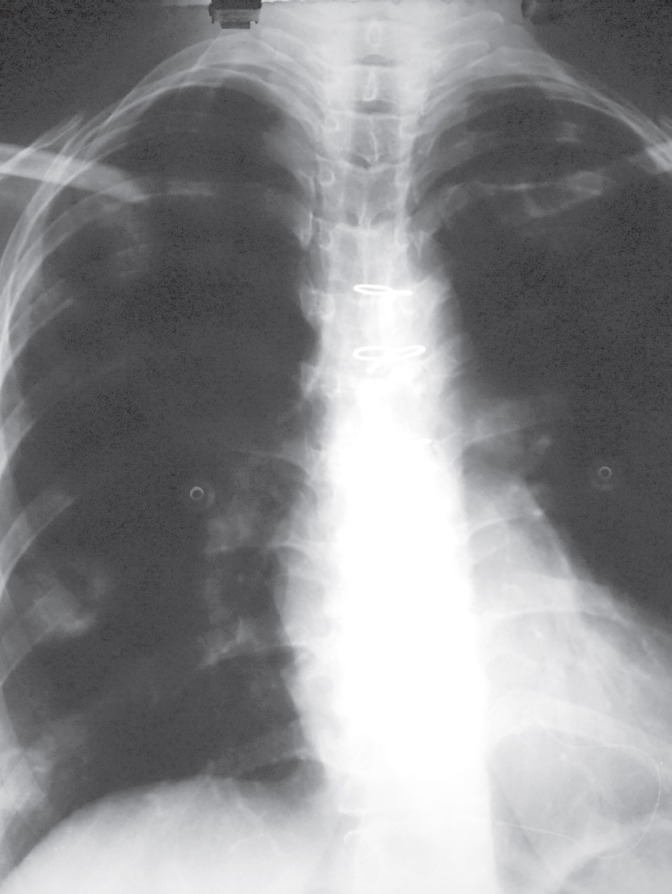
Normal chest x-ray at the time of initial presentation
